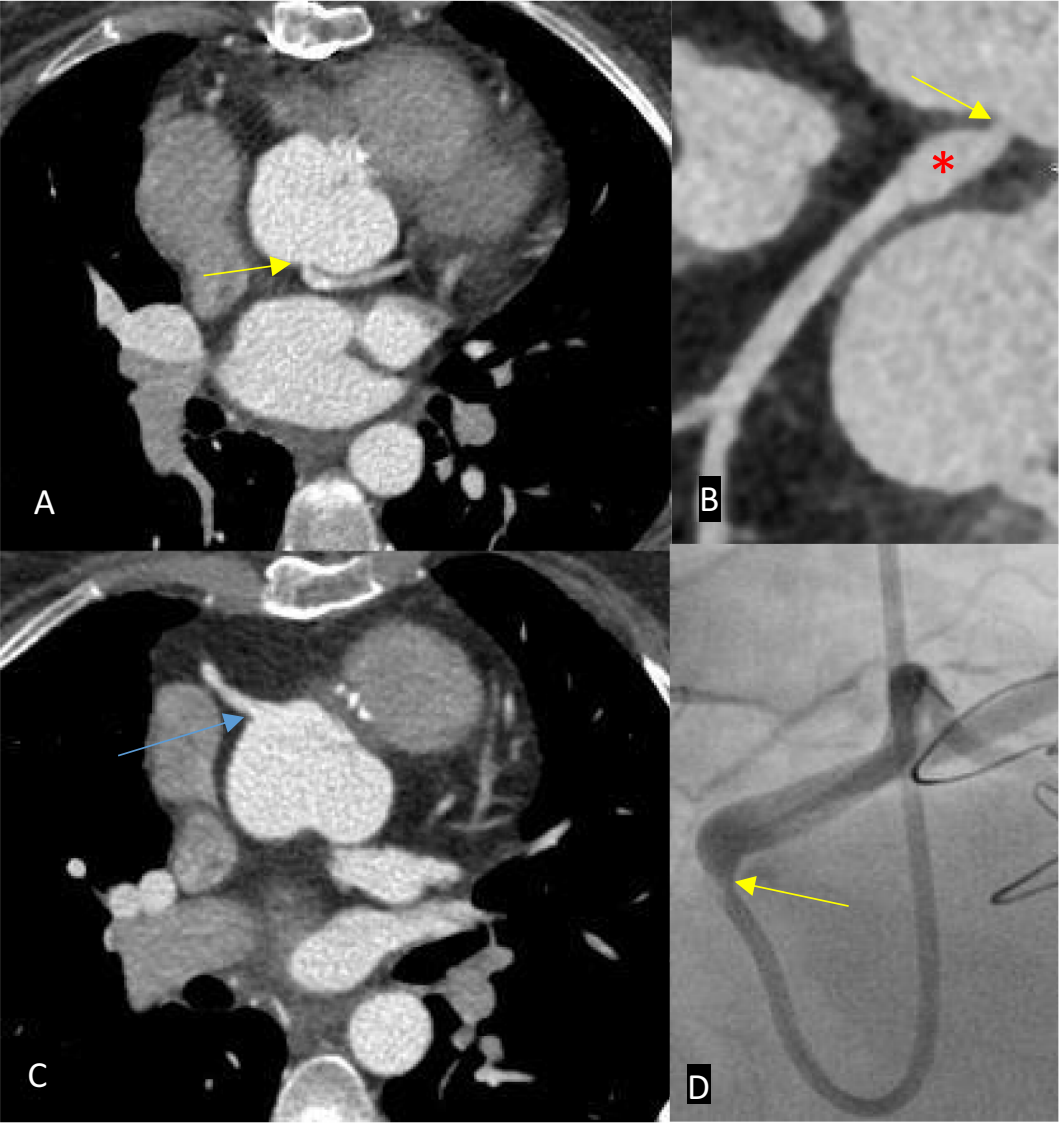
Select coronary CT angiography images (A, B, C) in a patient post-coronary artery translocation. A Axial view showing ostial stenosis of the LCA (yellow arrow). B Reconstructed view of the LCA showing ostial stenosis (yellow arrow) and post-stenotic dilatation (red asterisk). C Axial view showing the normal ostium of the RCA (blue arrow) for comparison. D Catheter angiogram of the LCA showing ostial stenosis (yellow arrow). LCA: left coronary artery; RCA: right coronary artery
